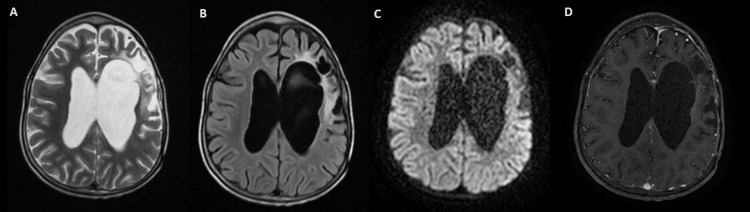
Three years post-treatment brain magnetic resonance imaging (MRI). (A) T2 axial image three years post-treatment showing hypersignal in the frontal and parietal lobes with enlarged left ventricle. (B) T1 axial image post-treatment showing frontal hypointense cavity with a surrounding hyperintense area, associated with a dilated left lateral ventricle. (C) Post-treatment diffusion-weighted imaging (DWI) axial image showing facilitated diffusion in the frontal post-treatment cavity. (D) T1 axial image post contrast injection showing no contrast enhancement in the frontal and parietal lobes.
